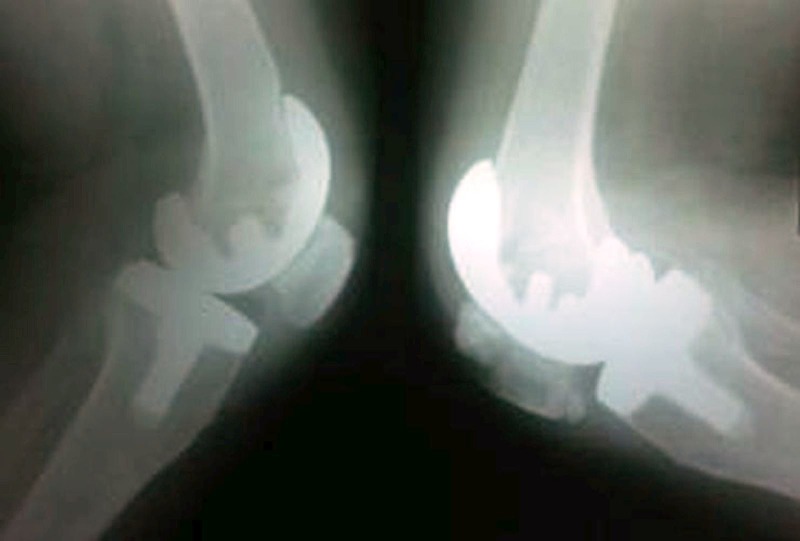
Lateral radiograph of a patient with a left patellar fracture three months after total knee arthroplasty
